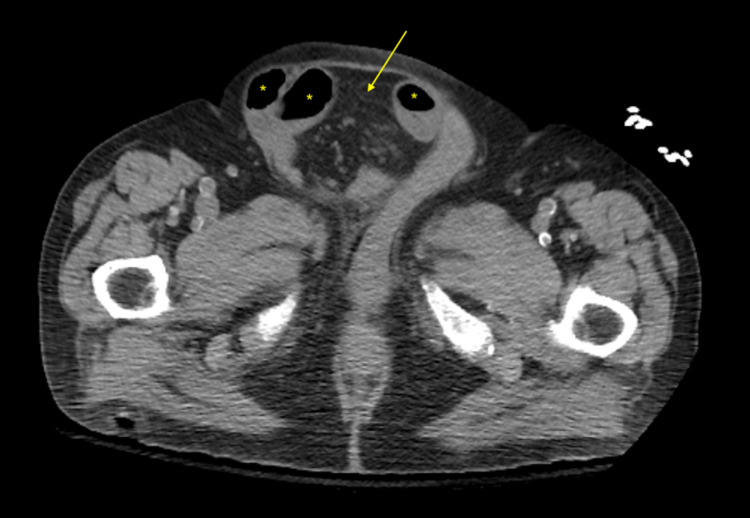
CT scan demonstrating a large right-side indirect hernia (arrow) with multiple loops of small bowel extending into the hernia defect (asterix), along with a direct hernia and small bowel distention throughout the abdomen. CT, computed tomography
